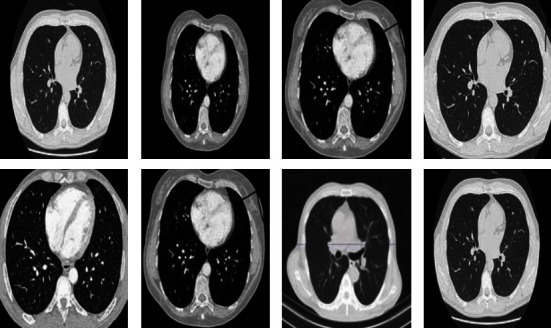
Chest-CT scan images (source: kaggle).
